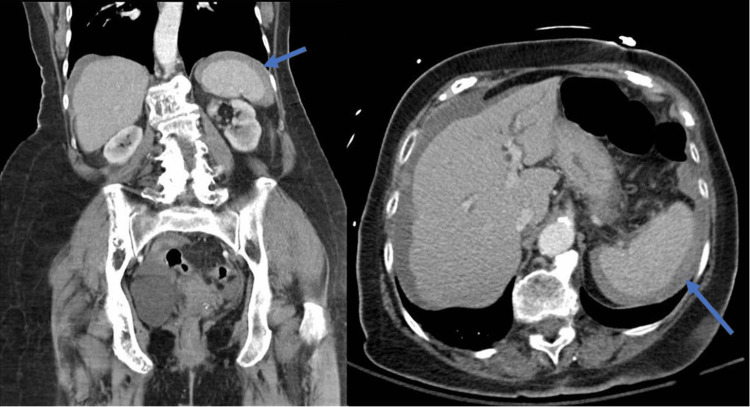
Computerized tomography (CT scan) of the abdomen and pelvis CT scan of the abdomen and pelvis showed moderate ascites and complex components at the left paracolic gutter and margins of the spleen suggestive of hemoperitoneum. A small hiatal hernia, an intrahepatic biliary duct with common bile duct dilation measuring 1.9 cm, and a 1.5 cm cystic lesion at the pancreatic body were also noted.
